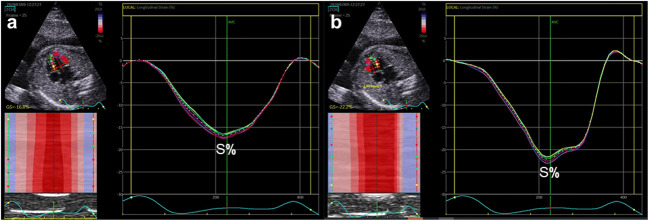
Global longitudinal strain analysis of left ventricle and right ventricle from the apical four-chamber view, with a graphic representation of average strain curves displayed as function of time for each of six segments. a Left ventricle. b Right ventricle. S%, peak systolic strain
